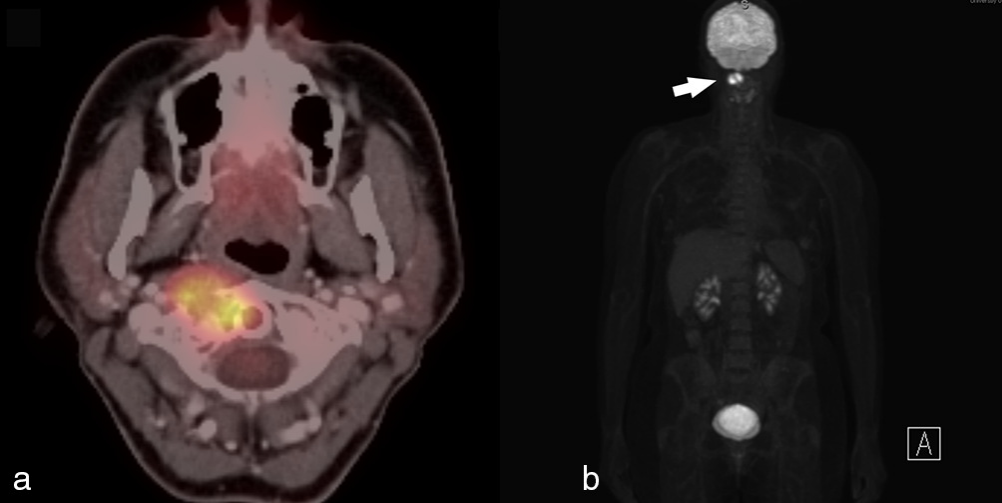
FDG-PET/CT imaging of a 49-year-old female with pigmented villonodular synovitis of the cervical spine. (a) Fused axial FDG-PET/CT image demonstrates the cervical spine lesion to be markedly FDG avid. (b) Coronal FDG-PET image demonstrates the C1/C2 lesion (white solid arrow), but there are no other suspicious foci of FDG uptake. Although seen with malignancy, FDG avidity is a marker of metabolic activity and is a non-specific finding that can also be seen with infectious, inflammatory and metabolic lesions. FDG, fludeoxyglucose; PET, positron emission tomography.
